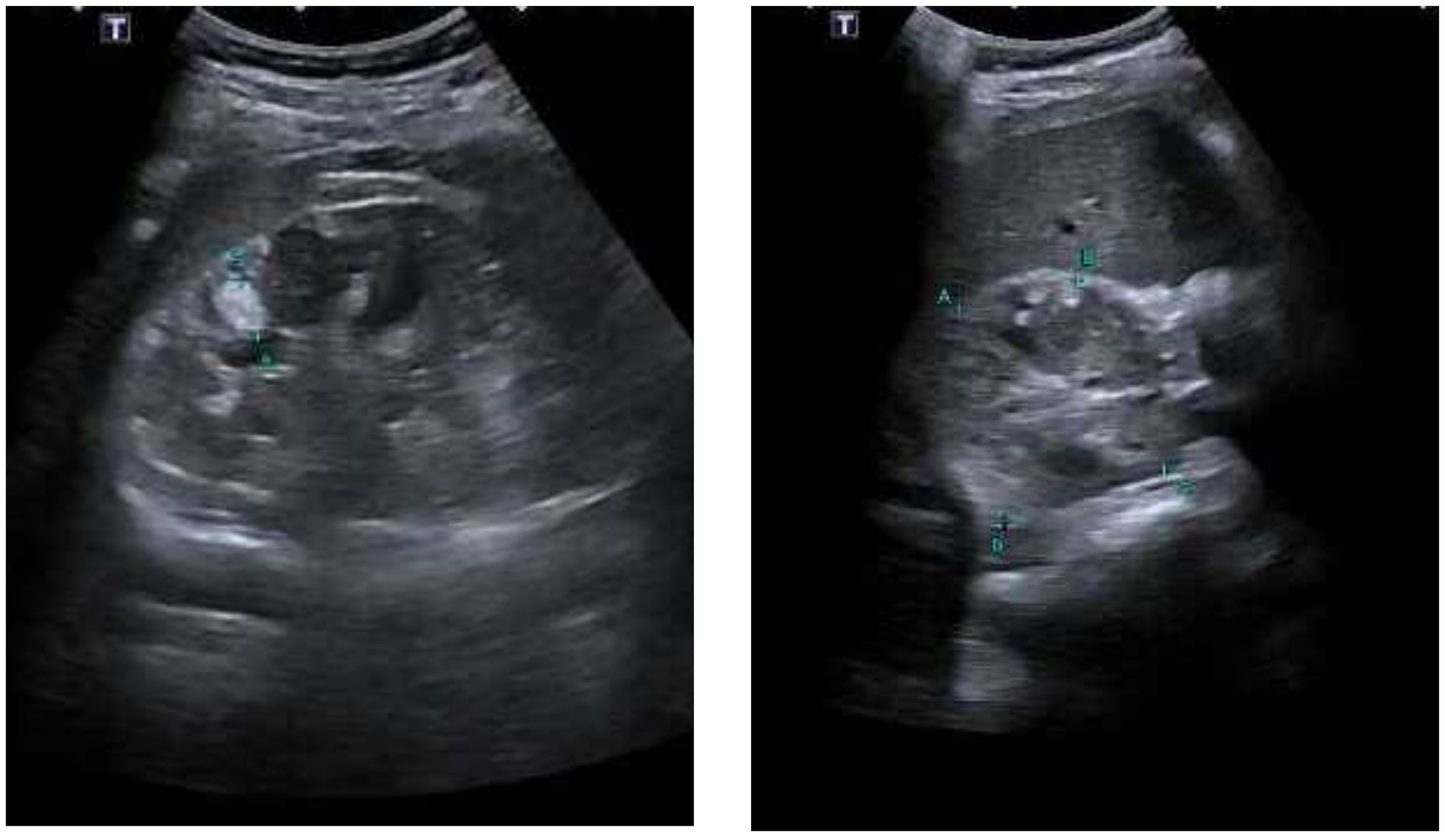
Bilateral renal ultrasound. Parenchyma displays multiple small rounded echogenic images distributed throughout, with sizes ranging between 12.5 mm, 10 mm, 7 mm, 5 mm, and 4 mm.
